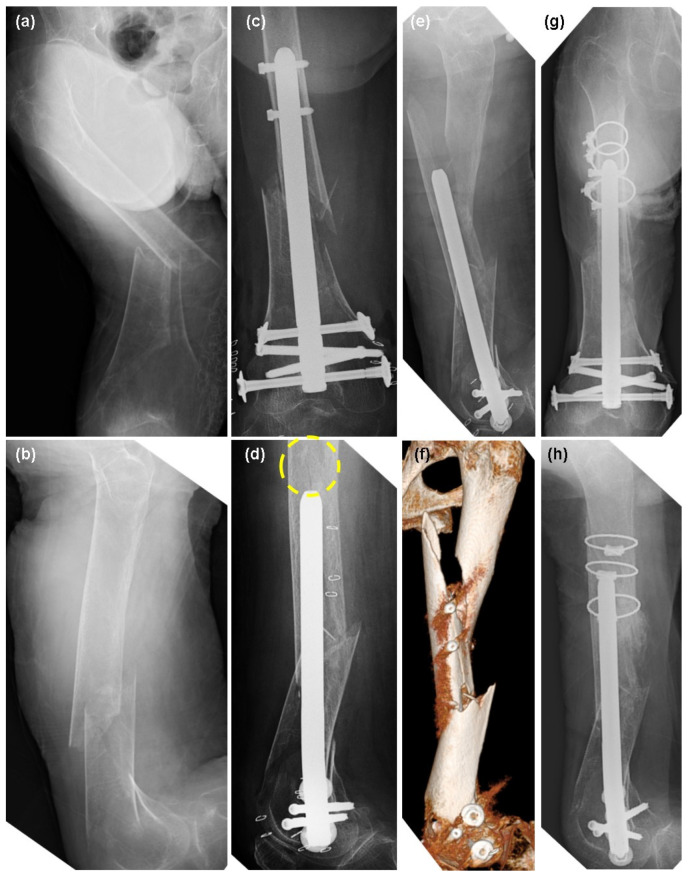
An 82-year-old female with a right supracondylar femoral fracture. (a) Preoperative frontal X-ray image of the distal femur. (b) Lateral image. (c) Postoperative frontal X-ray image. (d) Lateral image. The dotted circle shows the fracture line. (e) X-ray lateral image at one week postoperatively. A dislocated fracture was observed. (f) Postoperative CT image at one week postoperatively. The fracture was along the proximal advanced locking screws. (g) Re-fixation with cable wires (DePuy Synthes). Front image. (h) Lateral image.
